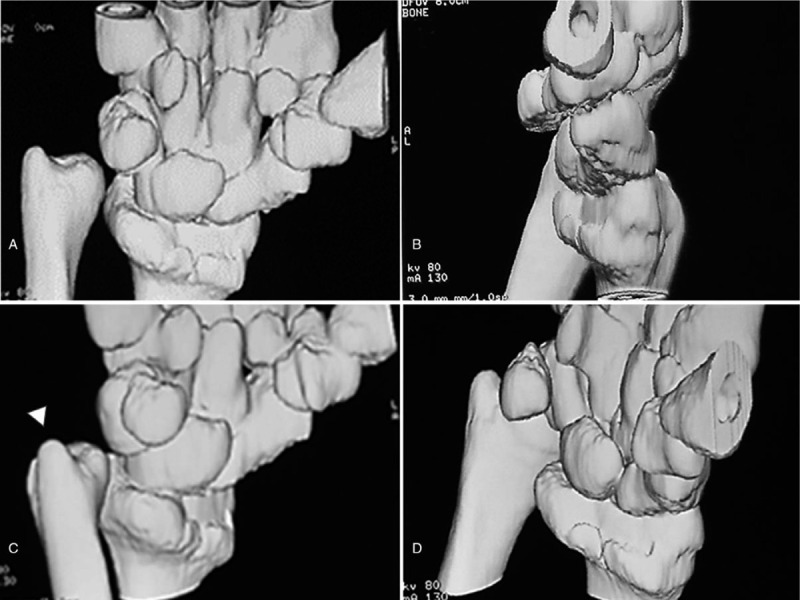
Three-dimensional computed tomography images of the distal forearm showing subluxation of the ulnar head to the posterior side of the sigmoid notch on the radius (arrowhead) and a volarly angulated distal radius.
